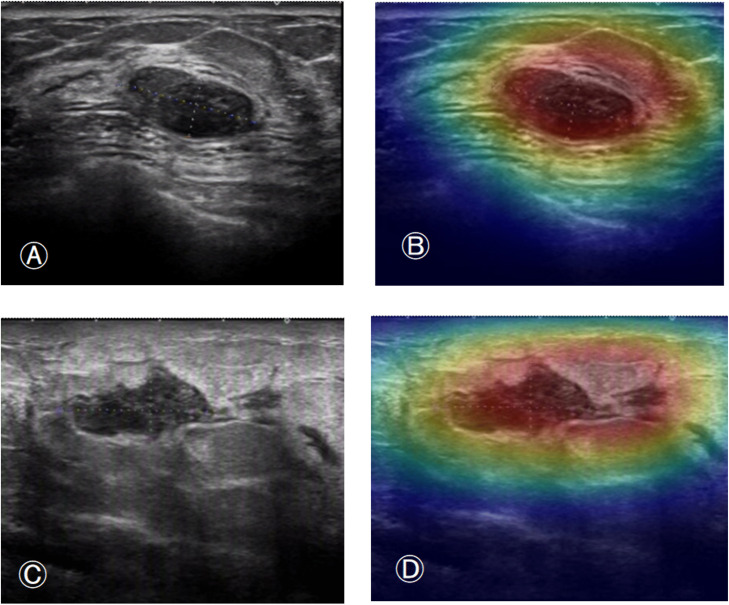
Figures A and C show B-mode ultrasound images of a fibroadenoma and an inflammatory masses, respectively, from the test set, and Figures C and D show the corresponding images after overlaying the thermograms using the pre-trained DenseNet121 model.
